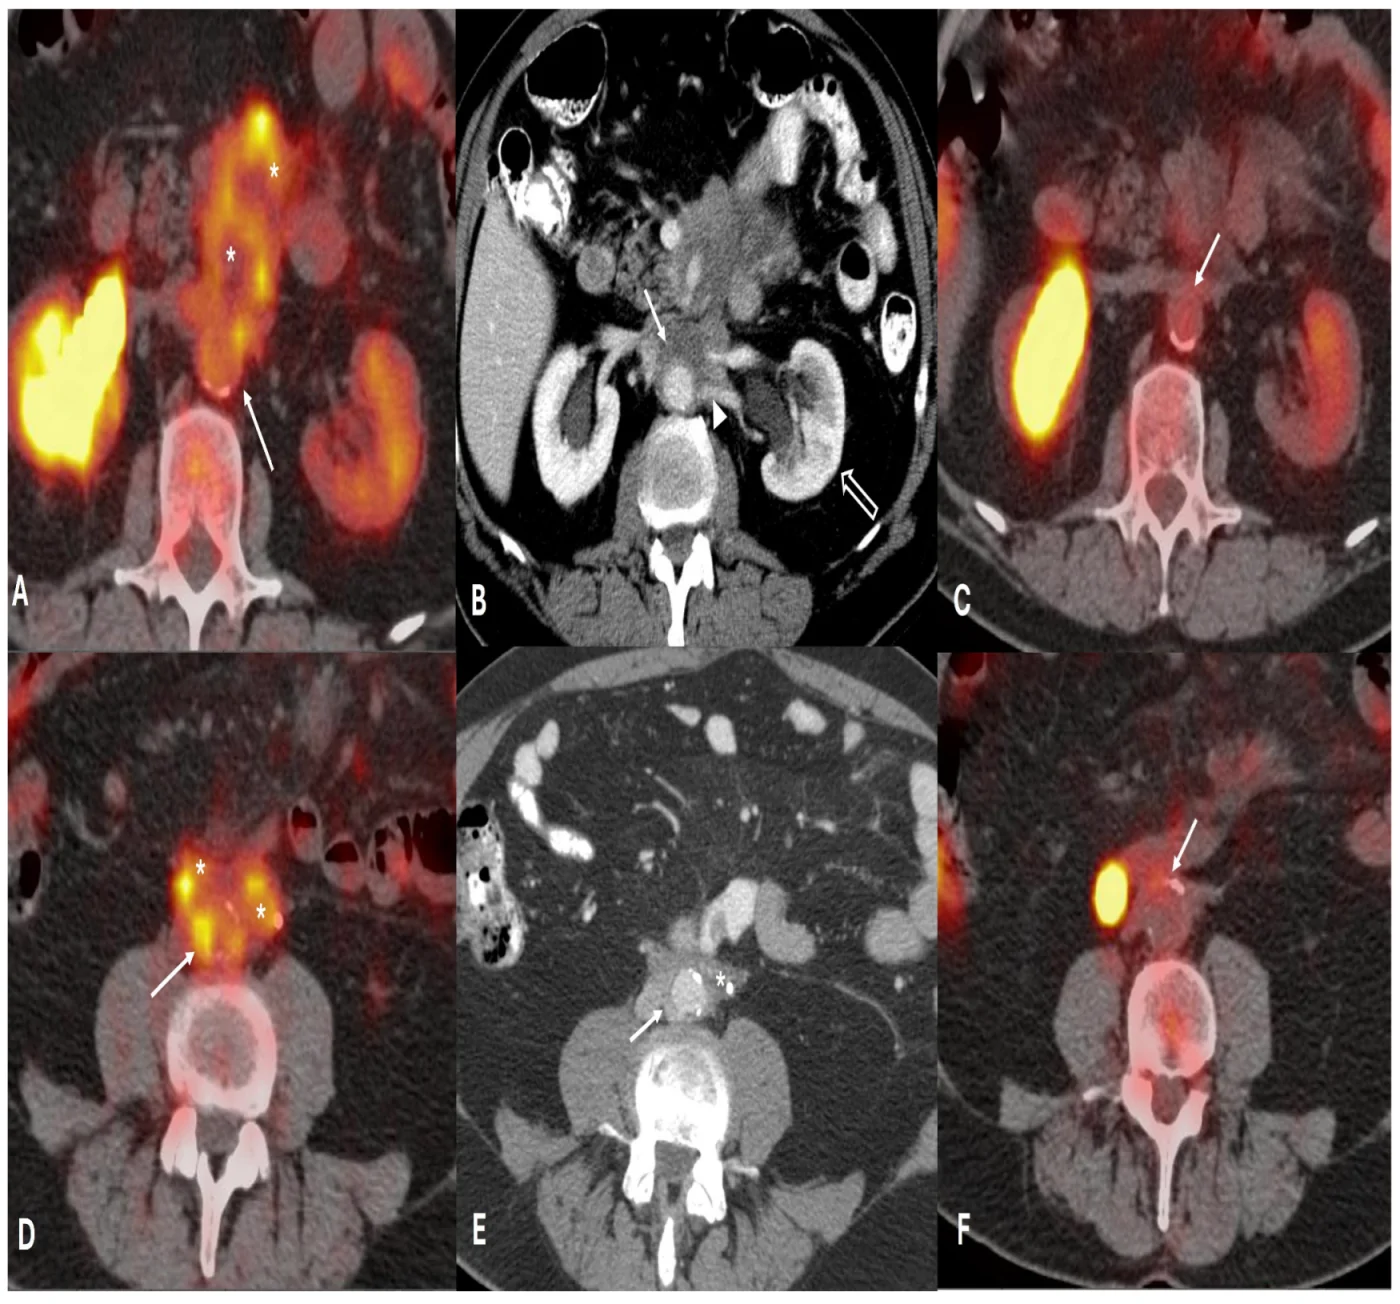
An example of known IgG4 disease with active vasculitis. Imaging before and after immunosuppressive therapy. Pretherapy images of fused axial F-18 FDG PET/CT at the level of the kidneys (A) and just above the bifurcation (D) and CT scan of the abdomen with oral and IV contrast at the same levels (B,E) showing tracer avid mural thickening to the abdominal aorta (arrows) and ostial portion of the left renal artery (arrowhead) associated to tracer avid mesenteric and retroperitoneal perivascular soft tissue mass (*) consistent with active vasculitis and surrounding inflammation. Please also note decreased perfusion to the left kidney (open arrow) secondary to ostial narrowing. Post therapy images of fused axial F-18 FDG PET/CT (C,F) showing near complete resolution of previously noted tracer avid vasculitis with surrounding inflammatory changes (arrows).
